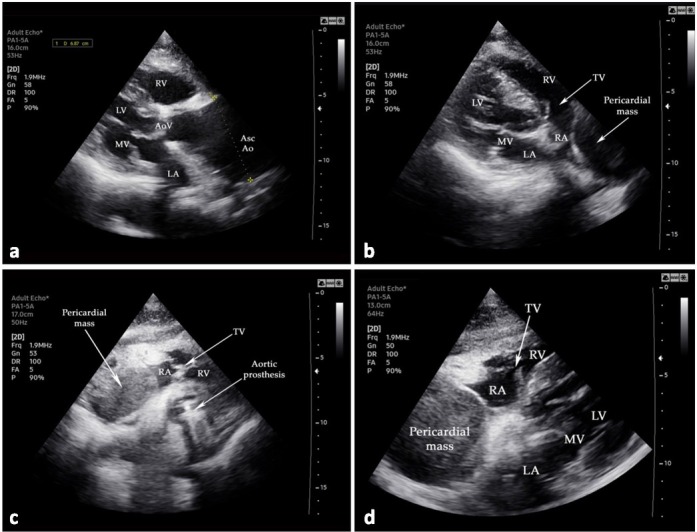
Transthoracic echocardiography examination: (a) the clear presence of the ascending aortic aneurysm (6.87 cm); (b–d) massive pericardial tumoral mass compressing the right atrium. Abbreviations: LA—left atrium; LV—left ventricle; MV—mitral valve; RA—right atrium; RV—right ventricle; SVC—superior vena cava; TV—tricuspid valve; AoV — aortic valve; AscAo — ascending aorta.
